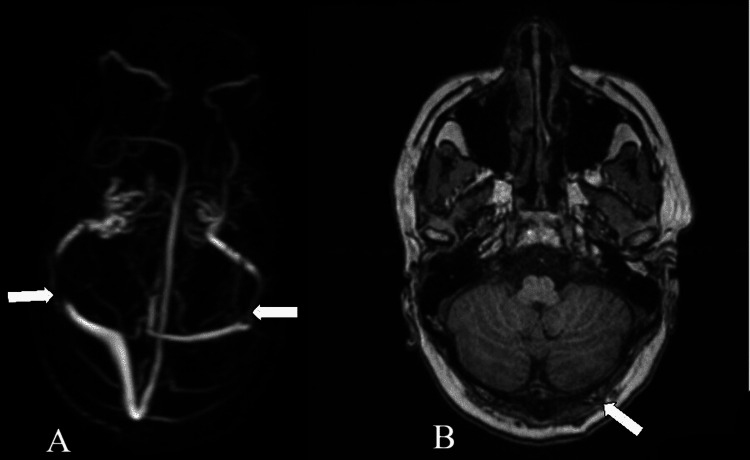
(A) Magnetic resonance venography showing a filling defect in the right transverse-sigmoid sinus junction and left transverse-sigmoid sinus junction showing luminal stenosis shown by white arrows. (B) Axial T1-weighted image showing increased signal demonstrating thrombosis in the left and right transverse-sigmoid sinus junction shown by the white arrow.
